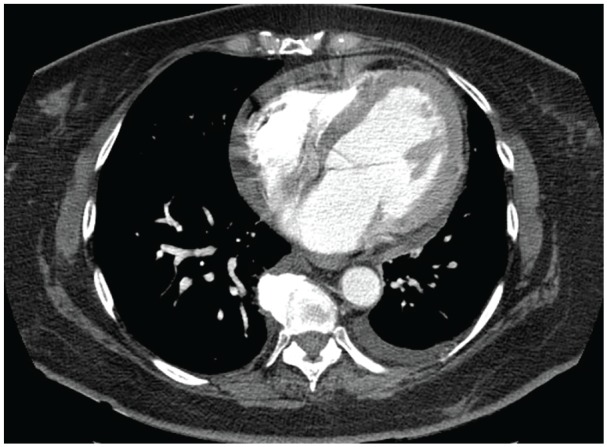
Axial contrast-enhanced computed tomography scan demonstrates small pericardial and left pleural effusions.
